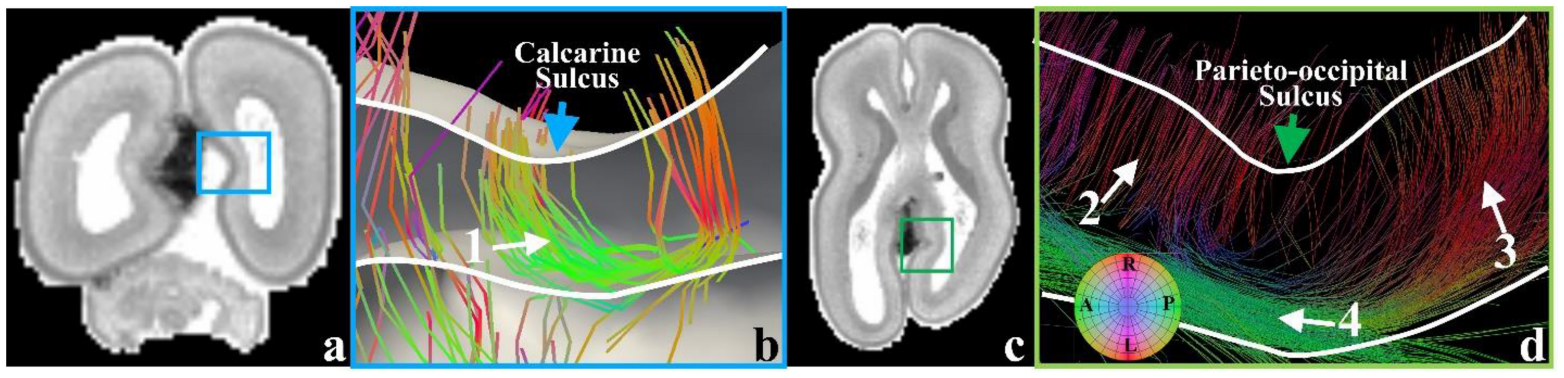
(a) A coronal T1-weighted MRI section of the 21 pcw fetal brain. The blue frame highlights the location of the calcarine sulcus; (b) Tractography fibers extracted from the calcarine sulcus. Arrow #1 indicates radial structure composed of RGCs and neuronal axons, coursing along the sulcal regions; (c) A transverse T1-weighted MRI section of the 21 pcw fetal brain. The green frame highlights the location of the parieto-occipital sulcus; (d) Tractography fibers extracted in the parieto-occipital sulcal regions. The green arrow highlights the sulcal fundus location on the cortical surface. Arrow #2 highlights RGCs. Arrow #3 indicates the radial structure, a mixture of RGC and neuronal axon. Arrow #4 highlights the parallel fibers beneath the sulcal fundus. White curves indicate the cortical plate boundaries.
